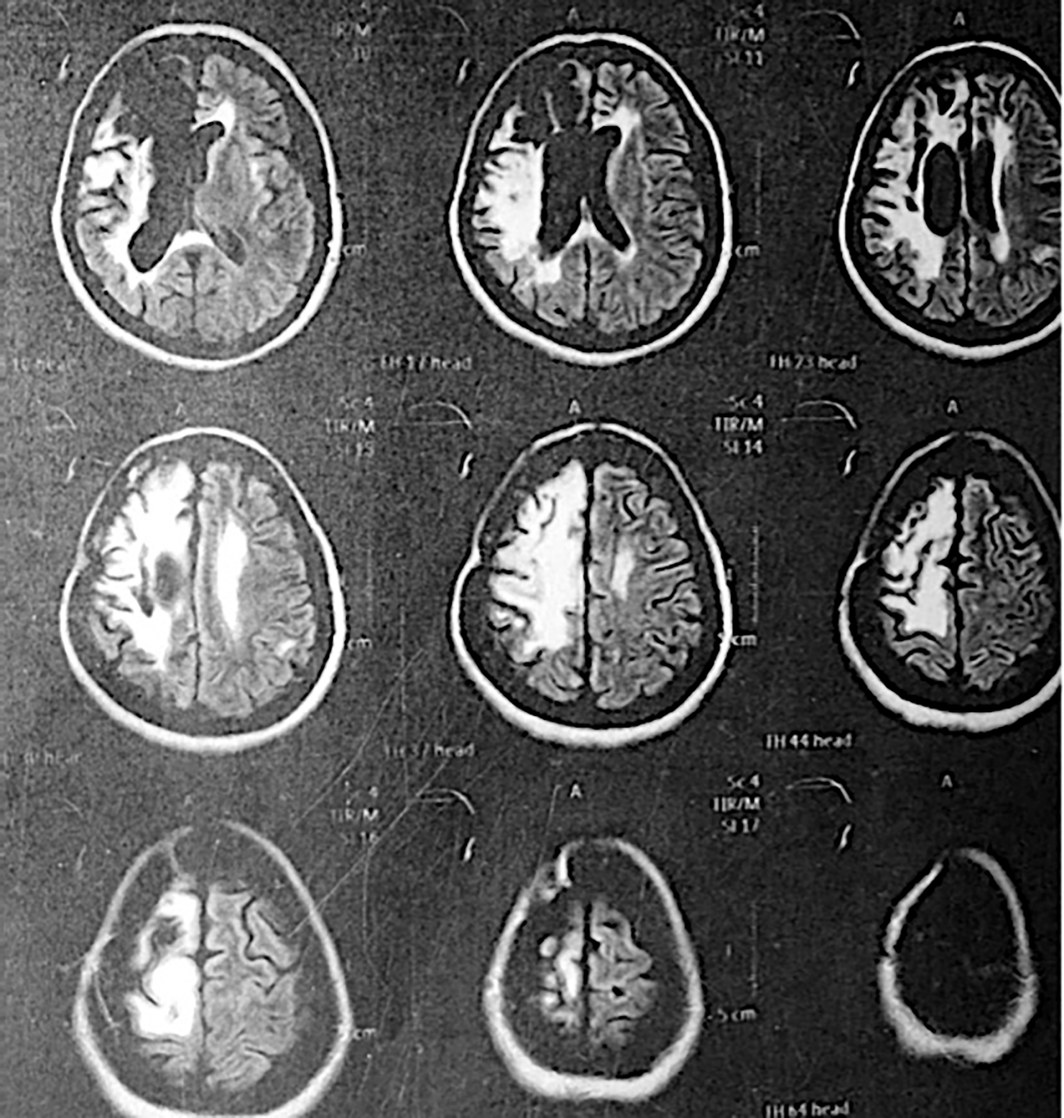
MRI of the patient years after surgery removal of the astrocytoma. An area of right frontoparietal encephalomalacia is observed with retraction of the frontal horn of the right lateral ventricle with slight perilesional edema.
